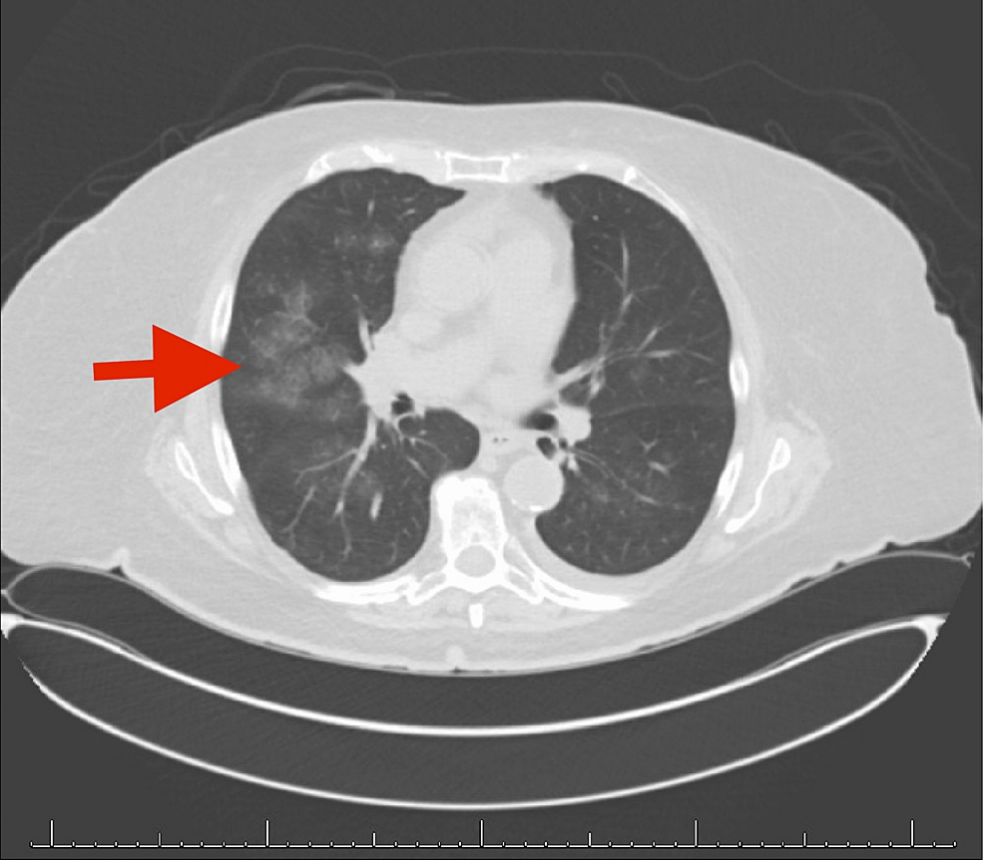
CT scan of the chest revealing pulmonary hemorrhage.
